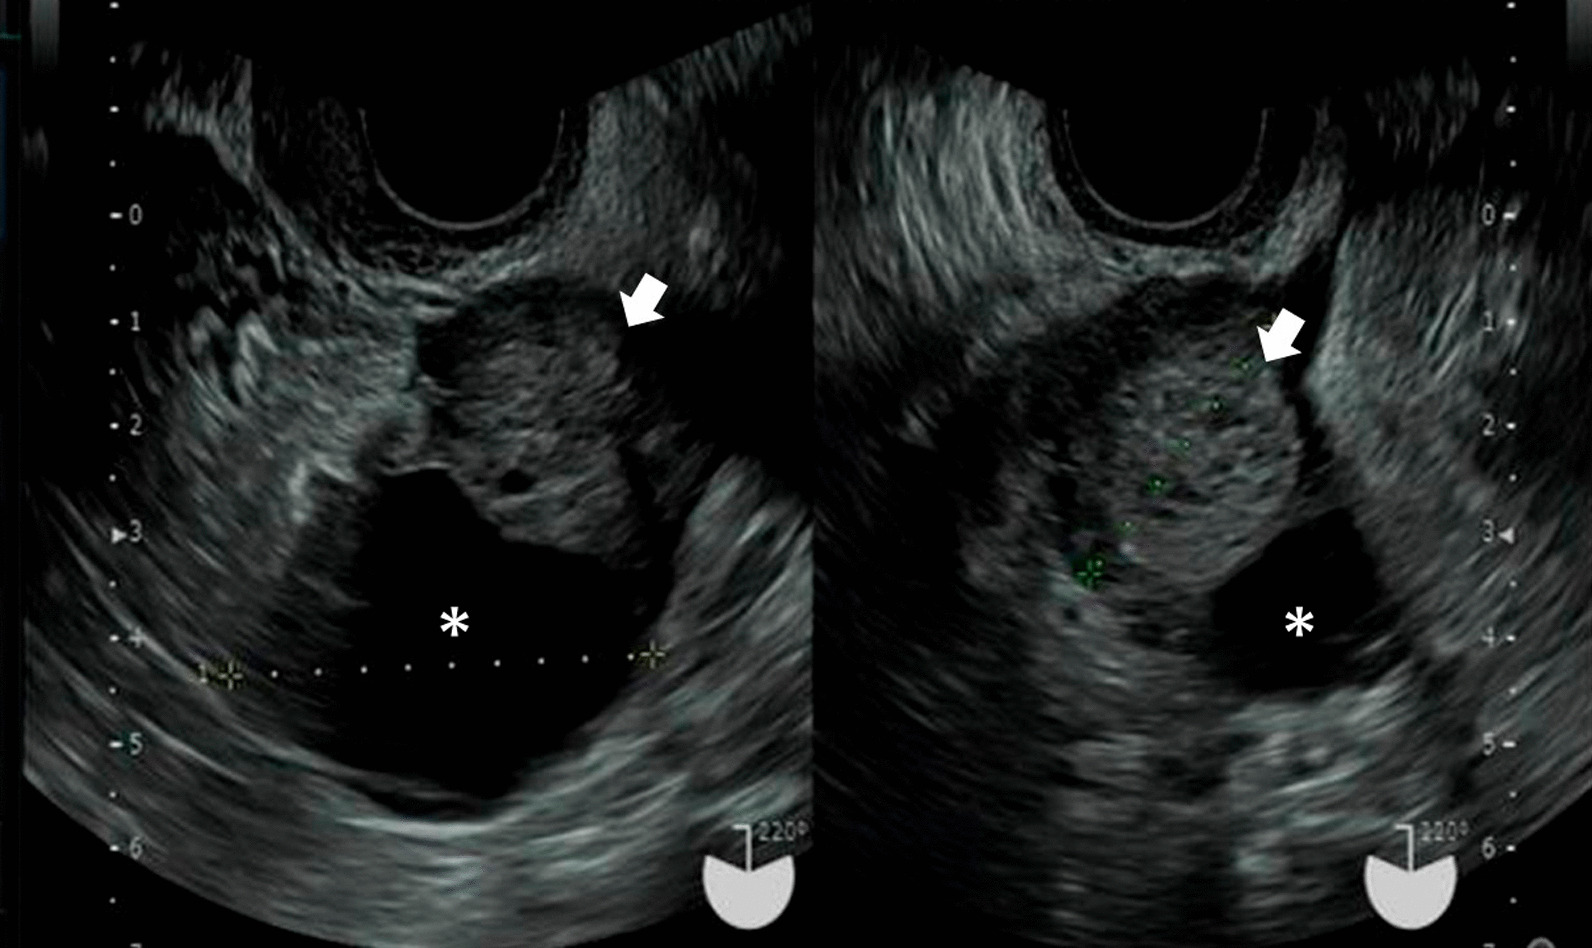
TVS of a cystic mass with solid component in the pelvis. Cystic and solid components measuring 60 × 35 mm in diameter localized at the left adnexa, suggestive of a lesion of the left ovary. *, a cystic mass with a maximum diameter of 4.8 cm. Arrow, a solid mass with a maximum diameter of 3.1 cm. *TVS* transvaginal ultrasound
